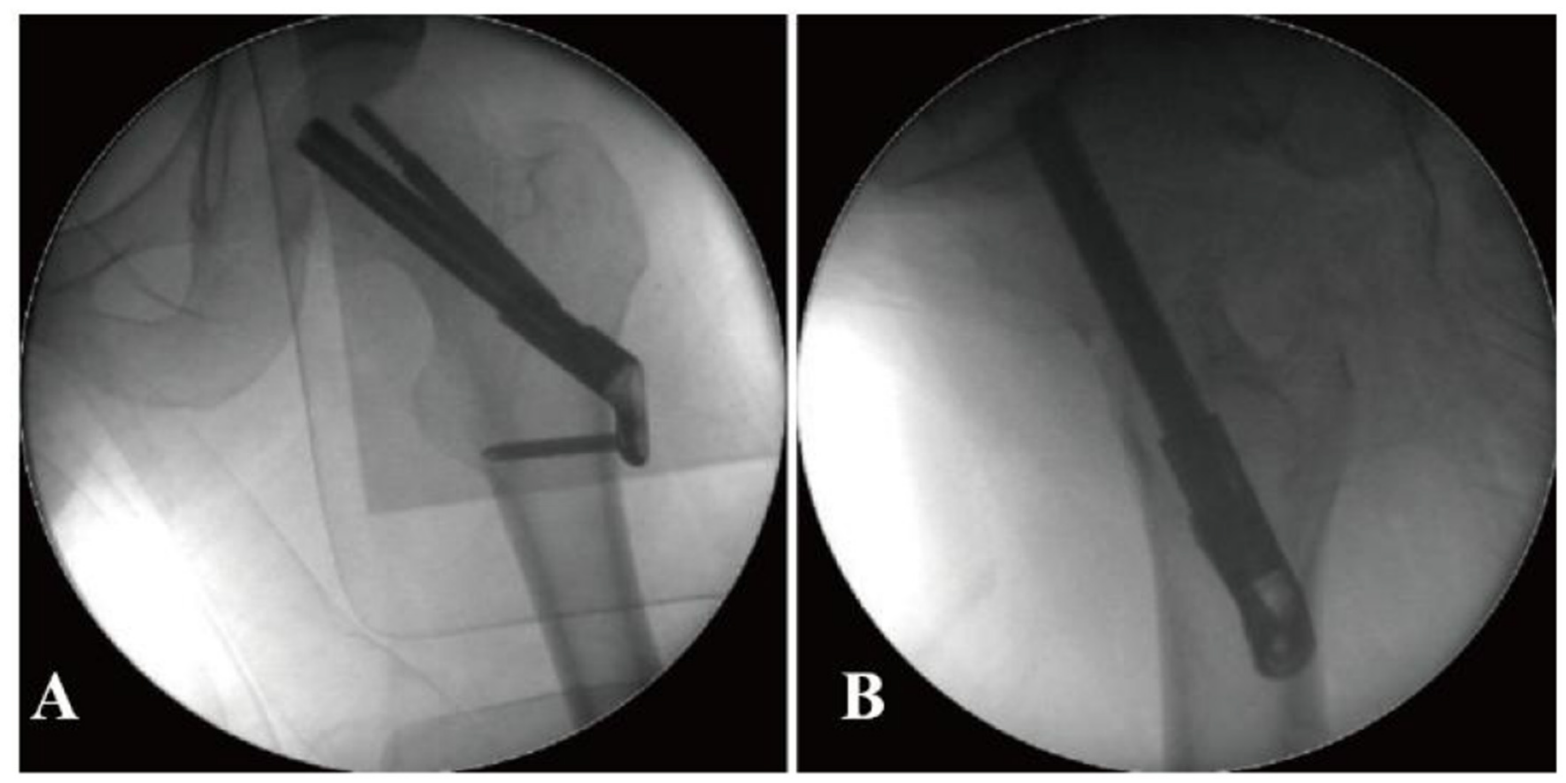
Radiographic and procedural details of FNS implantation in a 41-year-old male with a femoral neck fracture. (A, B) Anteroposterior and lateral views show the position of the implant. The tip of the FNS dynamic bolt was placed 5 mm from the femoral subchondral bone, followed by sequential insertion of the locking head screw and the anti-rotation screw. Due to impaction of the posterior cortex, the sliding distance of the FNS was preoperatively adjusted and limited to within 5 mm before final implantation to prevent excessive femoral neck shortening.
